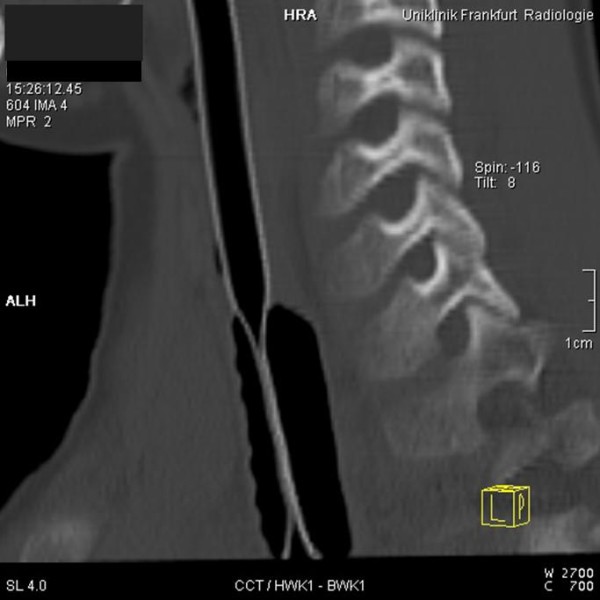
Computed tomography showing the sagittal plane of cervical spine and trachea. The cuff compresses the inner lumen of the endotracheal tube, thus leading to critical obstruction.
